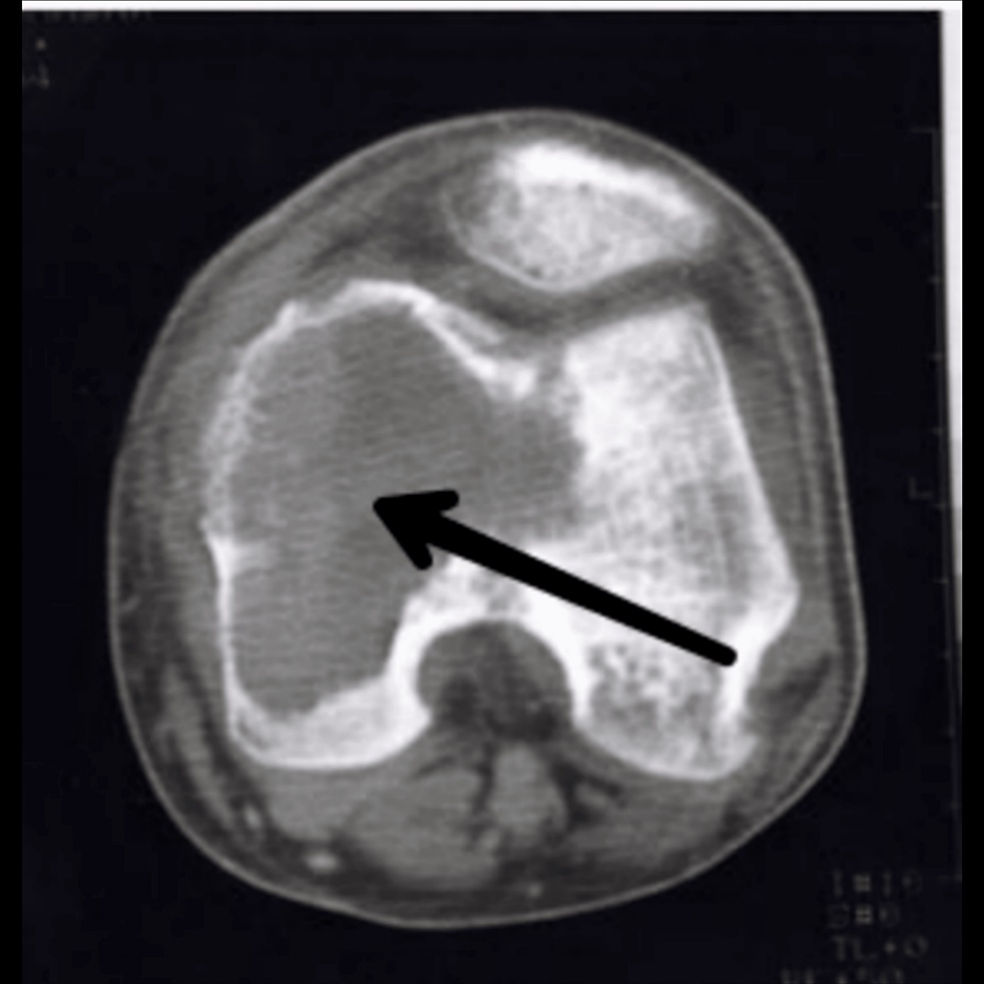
CT of knee joint showing GCTB in medial femoral condyle. Image taken by Yash Jha. GCTB: giant cell tumour of bone
